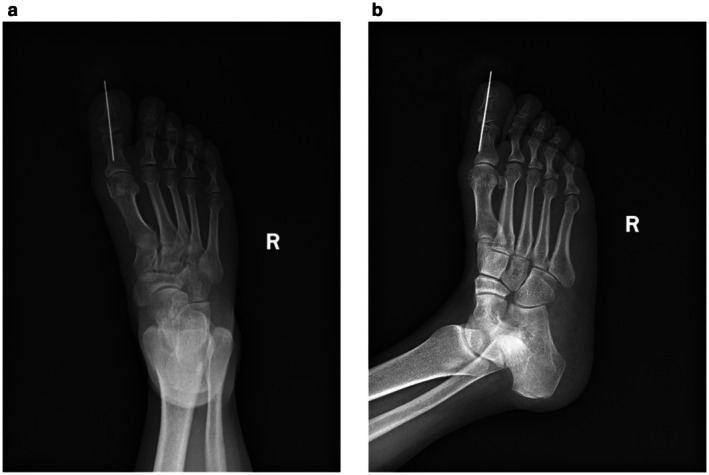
Radiographic evaluation of the right foot postoperatively revealed malalignment of the fracture due to impingement by a sesamoid bone.
